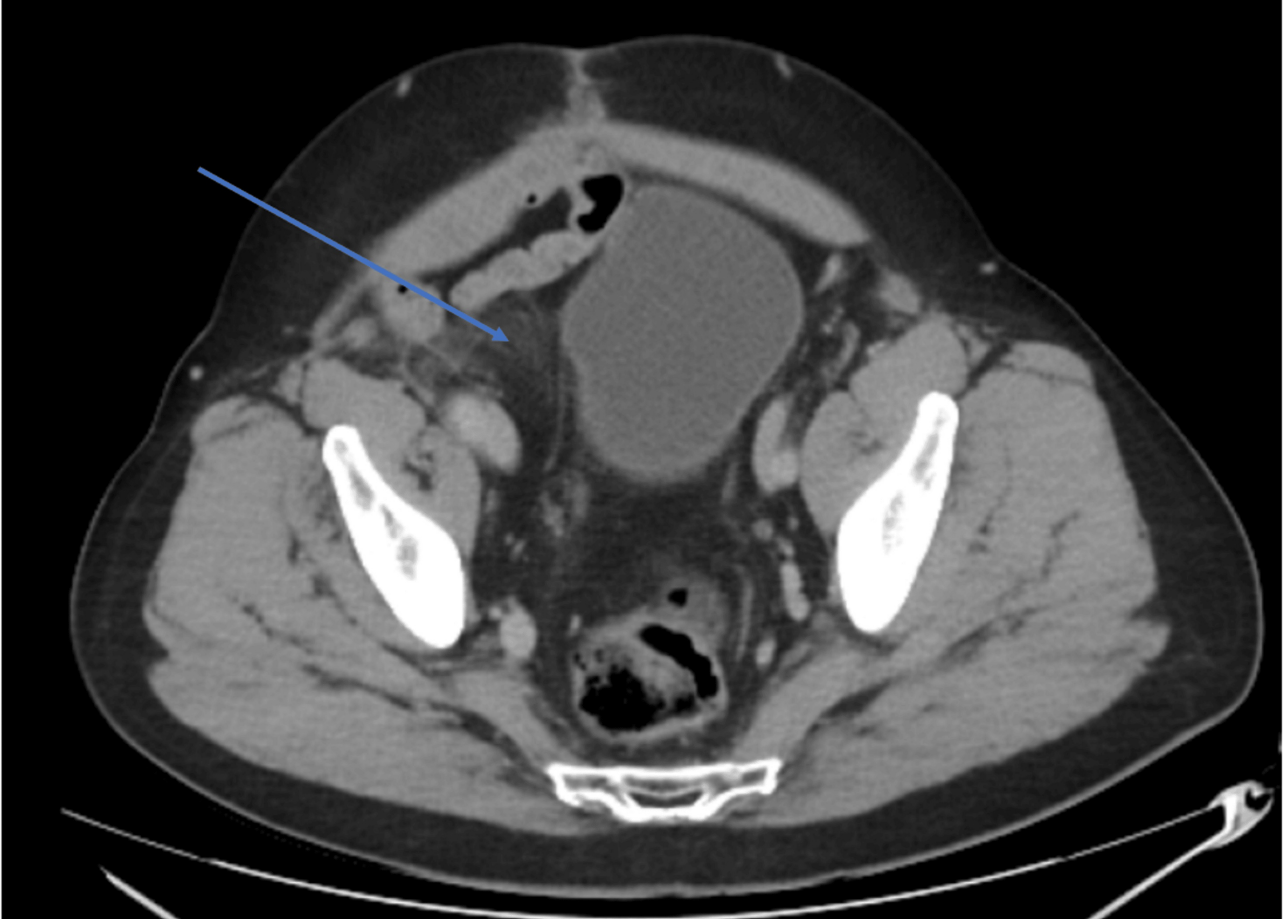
Case 2: CT taken following surgical resection showing a possible remnant of the original tumour in the right renal pelvis compressing the bladder.
